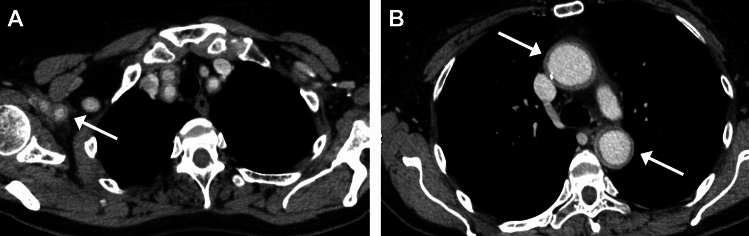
Large vessel involvement in giant cell arteritis. A 67-year-old woman with fever. Contrast enhanced CT shows wall-thickening and enhancement of the large arteries including the right axillary artery (arrow in A). The thickened aortic wall shows ‘double-ring’—like appearance (arrows in B)
